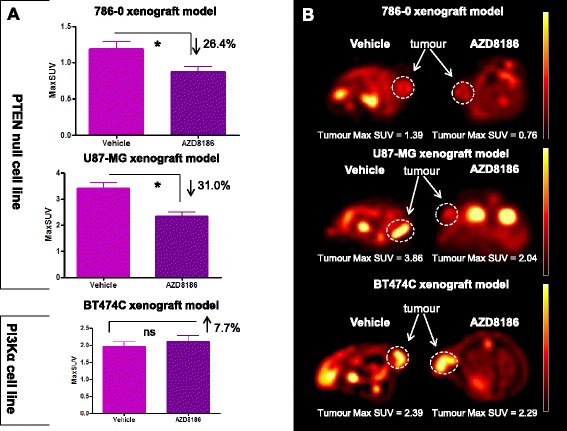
A single dose of AZD8186 (50 mg/kg) reduces 18F-FDG uptake 2 h after dosing in the PTEN null 786-0 and U87-MG xenograft models but not the BT474C PI3Kα model. a MaxSUV tumour 18F-FDG uptake following AZD8186 dosing (mean ± SEM). b Representative images from each cell line. 786-0: vehicle *n* = 10; AZD8186 *n* = 11. U87-MG: vehicle *n* = 8; AZD8186 *n* = 9. BT474C: vehicle *n* = 7; AZD87186 *n* = 8. p* < 0.05 unpaired *t* test
